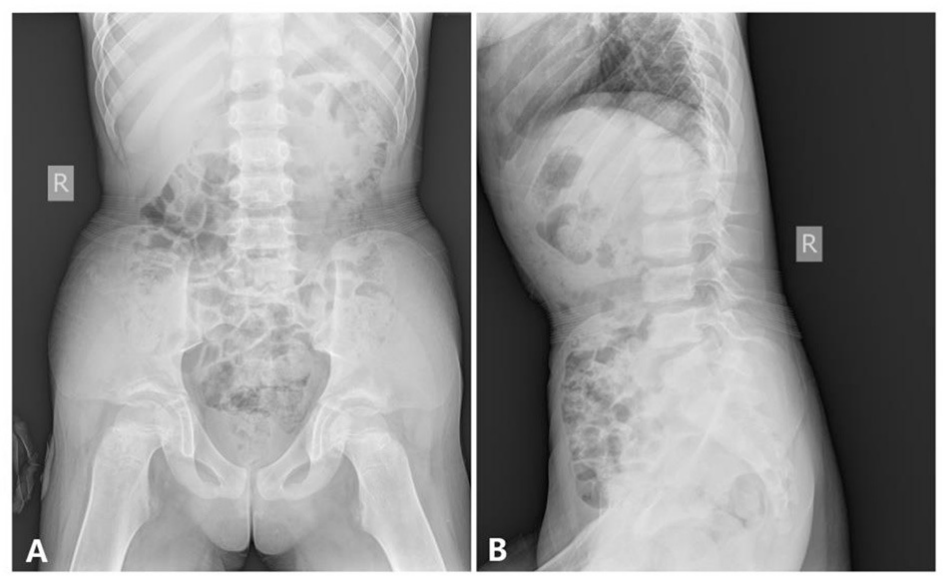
The x-ray radiography of the abdomen and the pelvis [age 6 years and 8 months, (A) anteroposterior film, (B) lateral film, shows].
